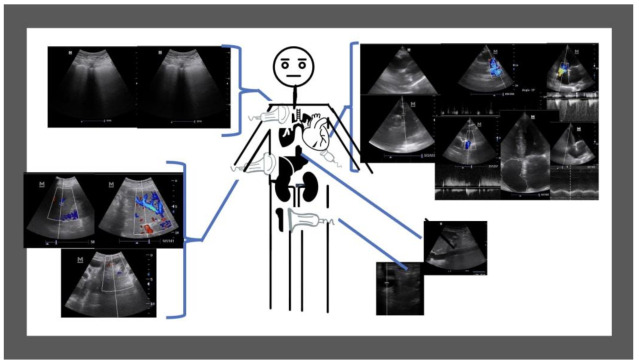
Representation of the ultrasound exam performed in all patients (*n* = 30).
